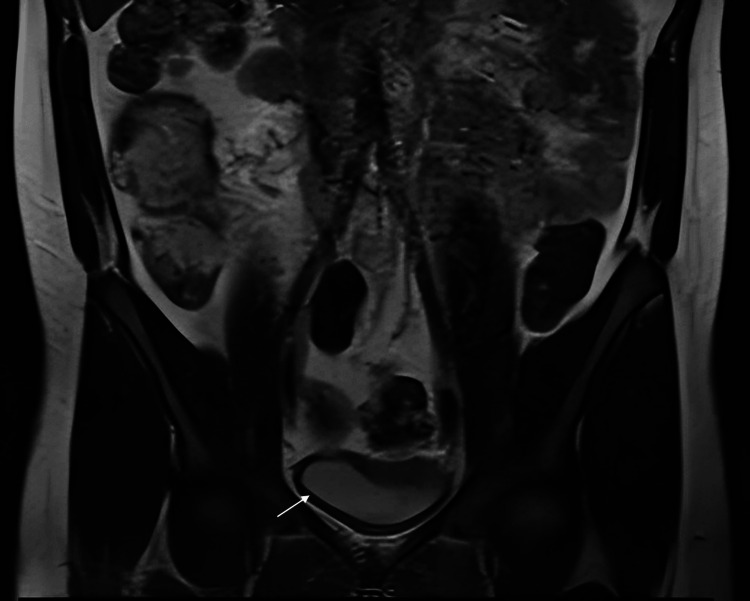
Coronal T2-weighted MRI of a patient with Crohn's disease, showing mild bladder wall thickening (arrow) and adjacent bowel changes suggestive of active disease, without evidence of an enterovesical fistula.
